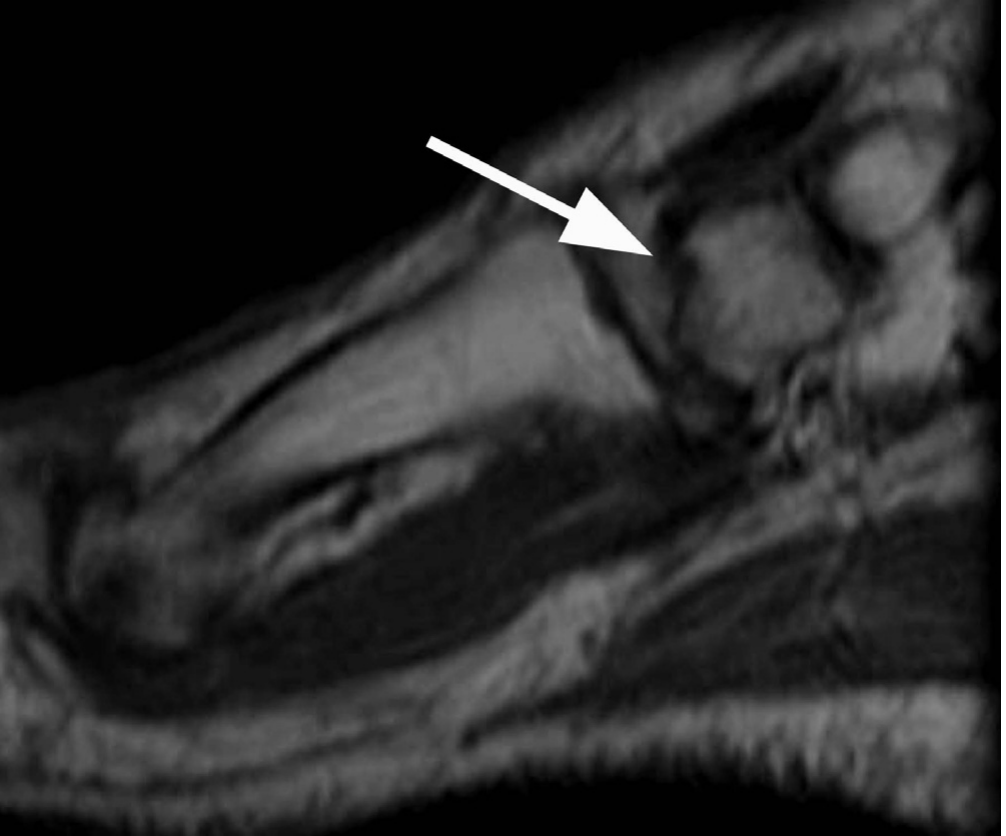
Sagittal T1 spin echo MR image (TR/TE = 700/9 ms) of case #3 demonstrates an oblique, hypointense fracture line (arrow) through the medial cuneiform, without significant displacement.
